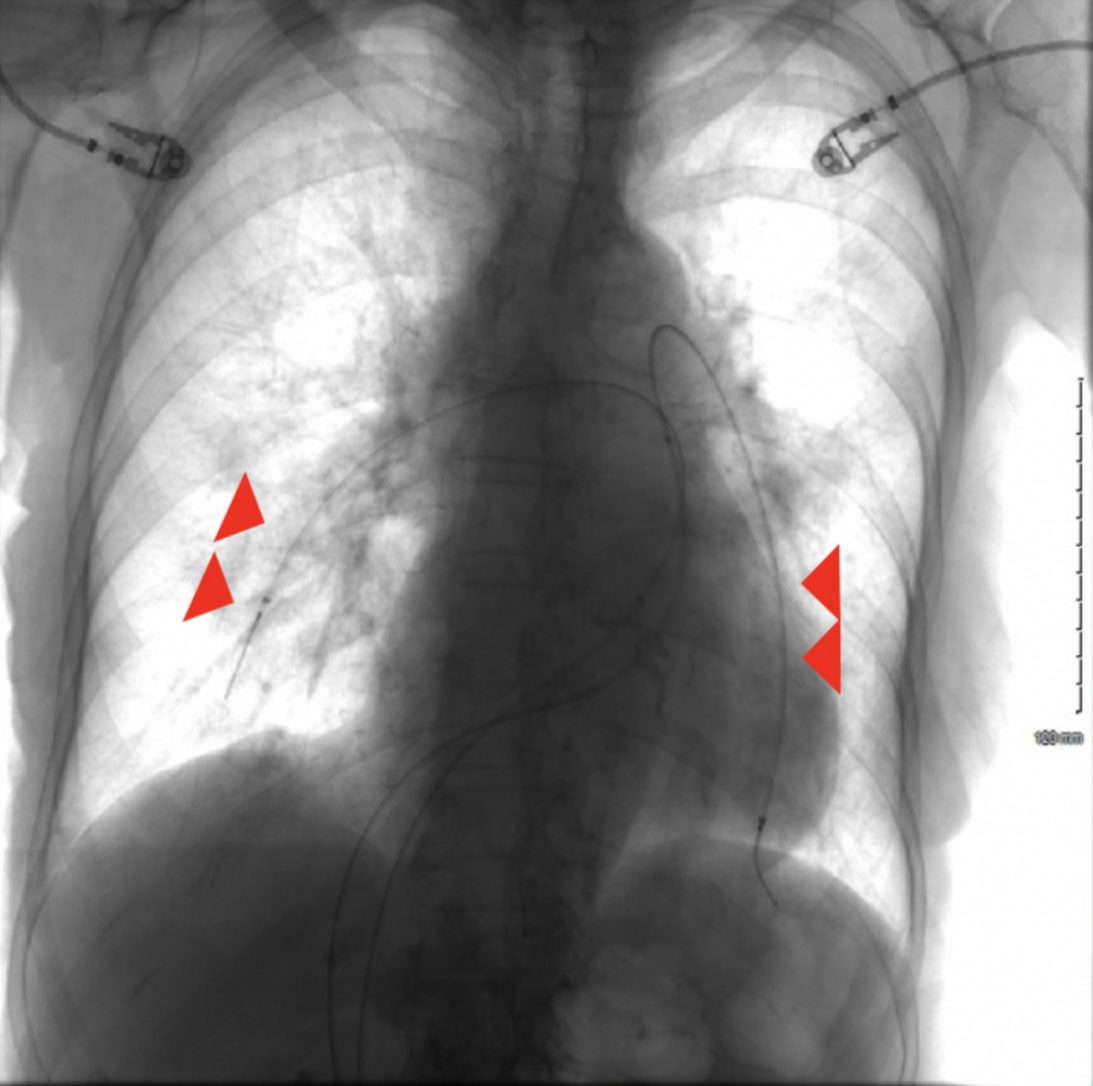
X-ray fluoroscopy demonstrating infusion catheters (arrowheads) placed for thrombolytic administration.
